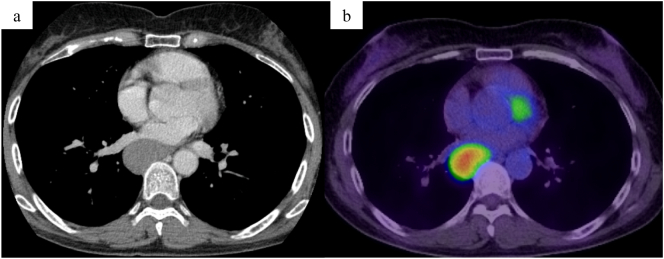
(a) CT scan showed the tumor with heterogeneous density and no metastasis in the lung and the liver. (b) Positron Emission Tomography CT revealed SUVmax 9.67 in the mediastinum on the right side of the esophagus.
